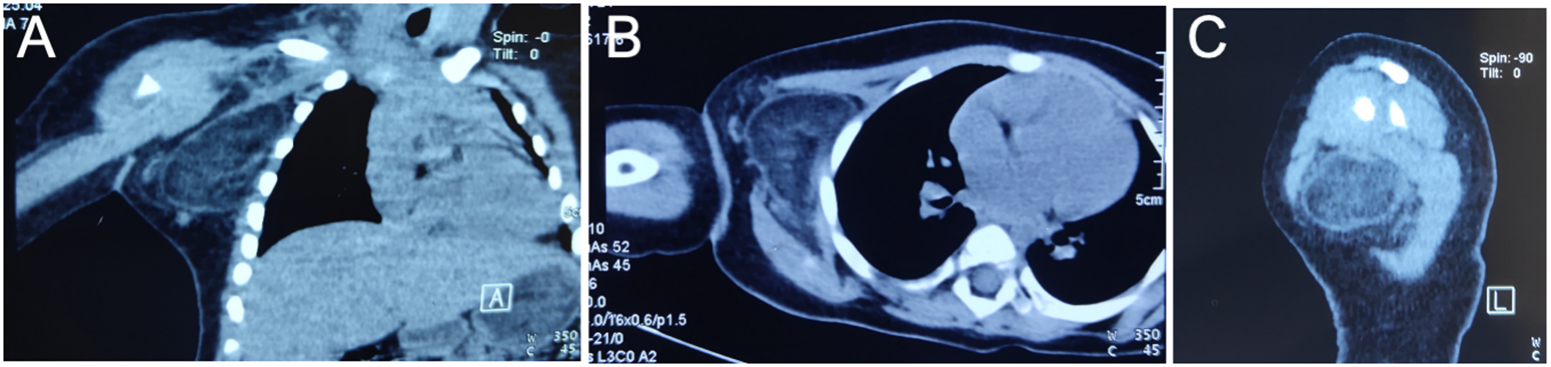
Coronal (A), transverse (B), and sagittal (C) plane CT images of the patient's upper extremity prior to excision. A circular low-density lesion was found in the right axilla, characterized by uneven density and clear edges. The CT value was approximately −58 HU, and the cyst wall was smooth.
